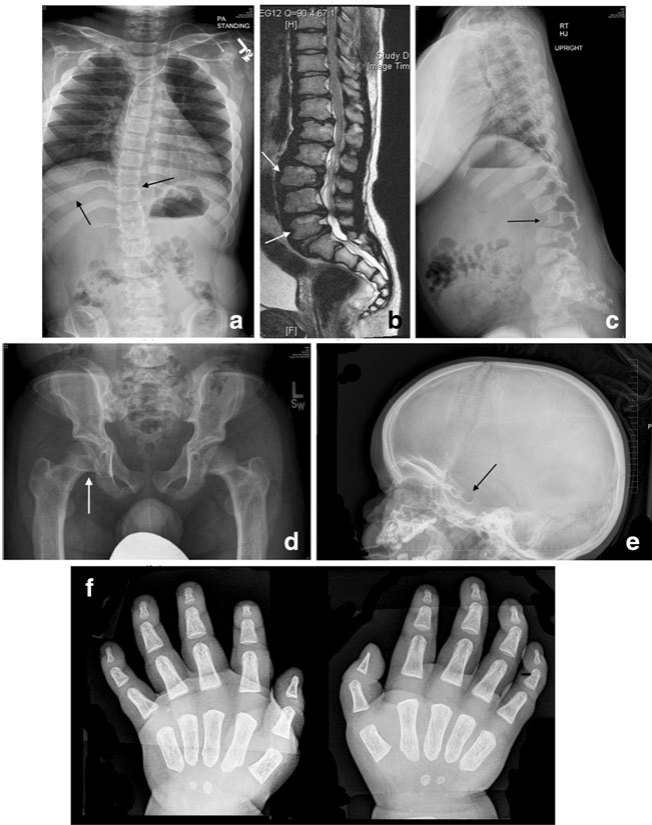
Skeletal manifestations of MPS II: a 33° scoliosis with coarseness of the bones and widening of the ribs in a 16-year-old patient with attenuated MPS II; b magnetic resonance imaging demonstrating mild thoracolumbar kyphosis and typical anterior vertebral body deficiency in a 16-year-old patient with attenuated MPS II; c significant thoracolumbar kyphosis in a 4-year-old patient with severe MPS II; d caput valgum and mild femoral head uncoverage in a 9-year-old patient with severe MPS II; e J-shaped sella in a 21-month-old patient with MPS II; f severe delay in maturation compatible with 3–6 months of age, mild proximal tapering of the metacarpals, and minimal slenderness of the distal phalanges in a 21-month-old patient with MPS II. Images a–d reproduced with permission from White et al. [45]
